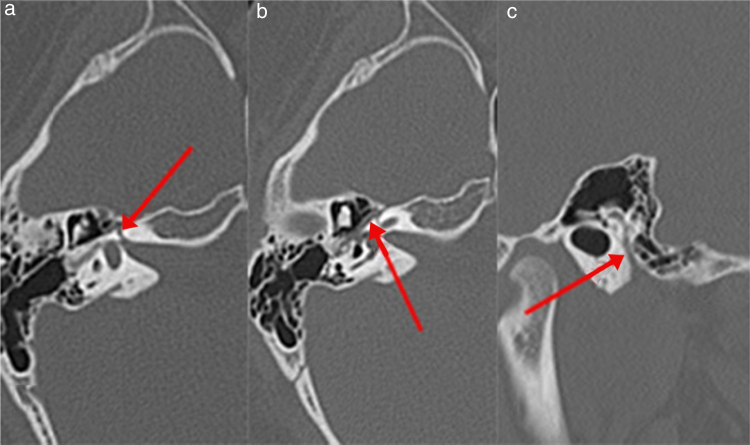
CT images of the left temporal bone of a 12 year-old girl with left Bell's palsy. The arrow indicates the facial nerve canal. (A) Labyrinthine segment, (B) tympanic segment, (C) mastoid segment.
